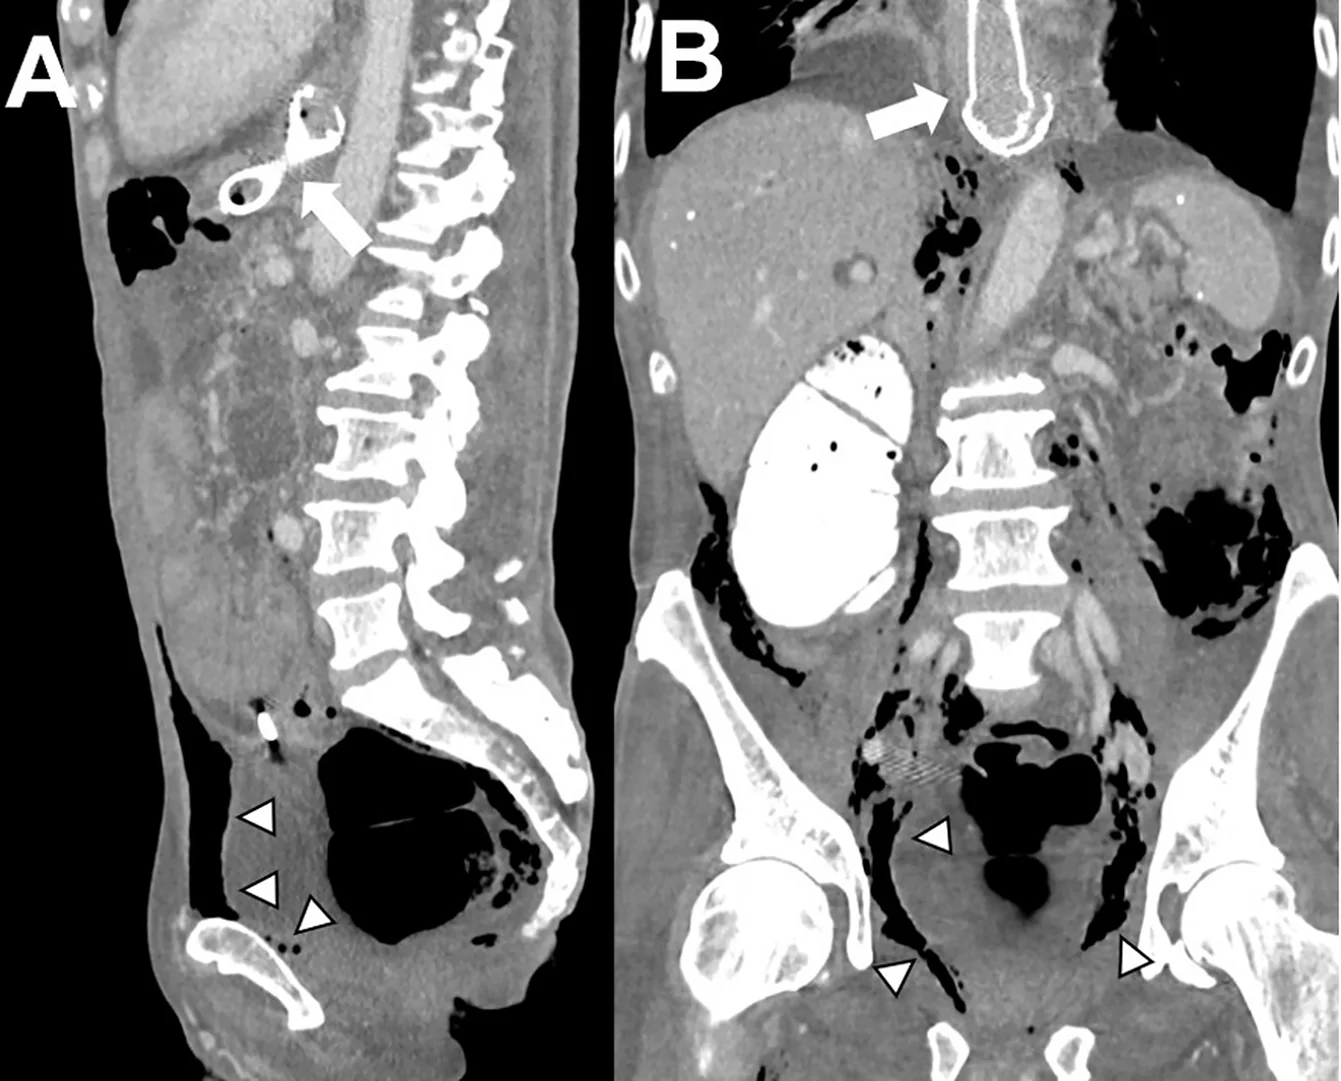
Sagittal and coronal reformatted CT images demonstrate free air in the space of Retzius and paravesical spaces (arrowheads in A and B) secondary to complicated esophagojejunal stenting (arrows). Note the air densities adjacent to the stent with inferior extension to the level of the space of Retzius
